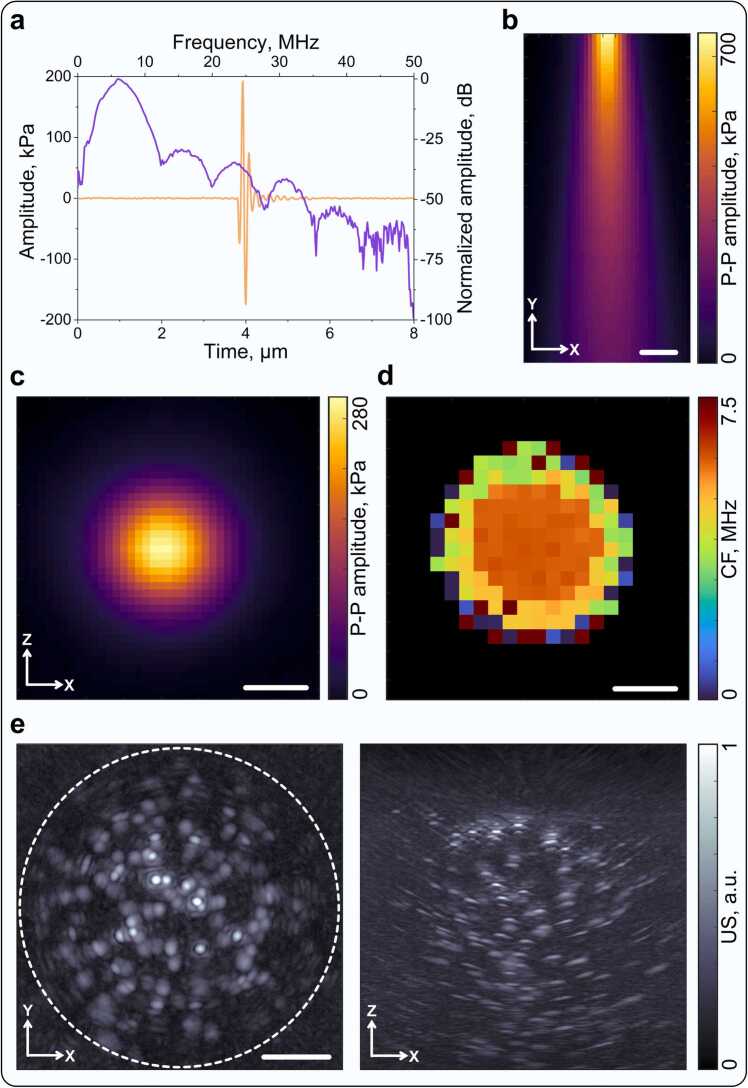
a) Characterized signal from the single-element transducer used for microbubble excitation in the experiments: time-domain signal and its Fourier transform. b) Axial peak-to-peak (P–P) pressure distribution measured at distances of 15–55 mm from the transducer. Scalebar: 5 mm. c) Transverse section of the peak-to-peak pressure amplitude at 40 mm distance from the transducer. d) Central frequency (CF) distribution within the ultrasound (US) beam at 40 mm distance from the emitting surface. Scalebars: 5 mm. e) Pulse-echo US image of 90 µm black polyethylene spheres embedded in agar with an optical scattering layer, showing the effective field of view (FOV) in two projections. Effective imaging circle: 10 mm diameter. Scalebars: 2 mm.
